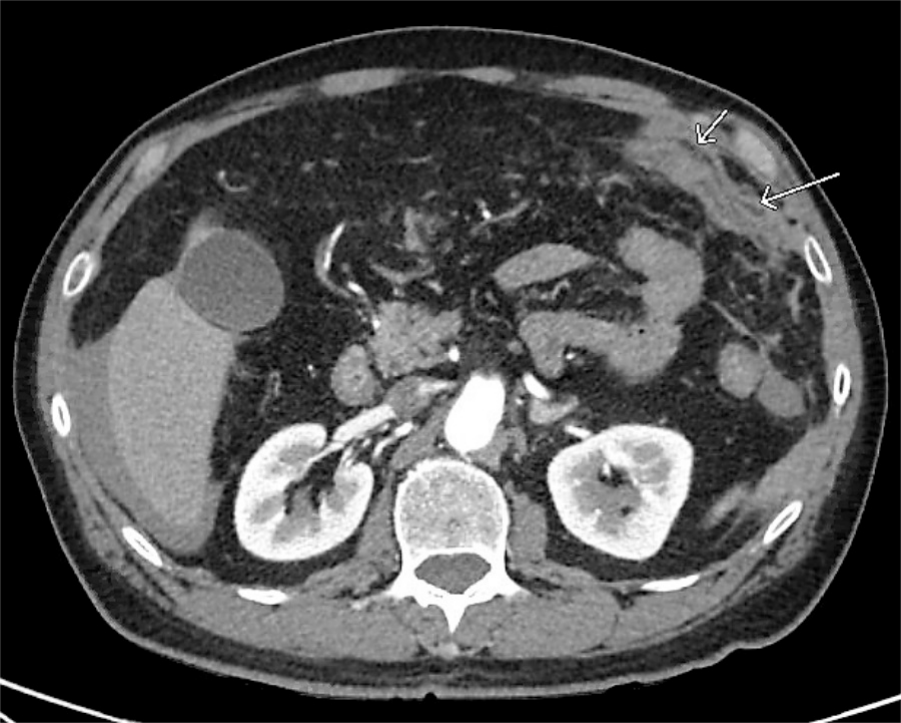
Transverse section on CT abdomen showing soft tissue attenuation with surrounding fat stranding consistent with hematoma CT, computed tomography
